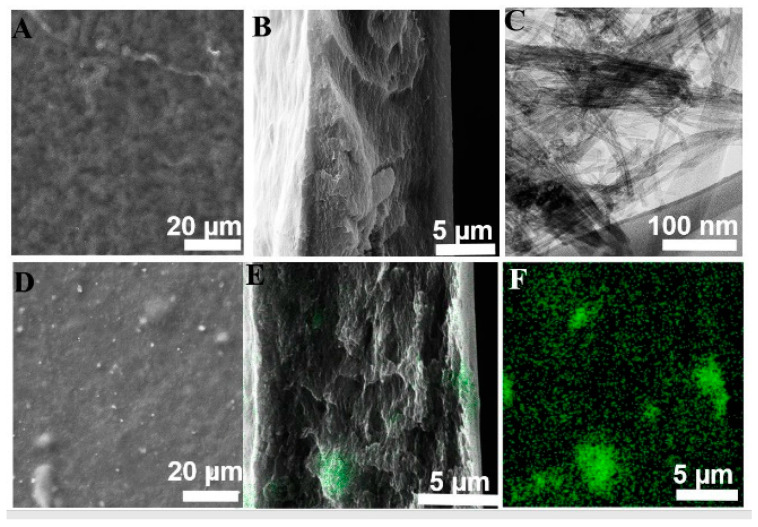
(A,B) Surface and cross-section of CTA-CNT; (C) TEM image of CNT@TNT; (D) SEM of the surface view of CTA-TNT@CNT; (E) EDS overlayed cross-section image of CTA-TNT@CNT; (F) EDS showing Ti distribution of (E).
